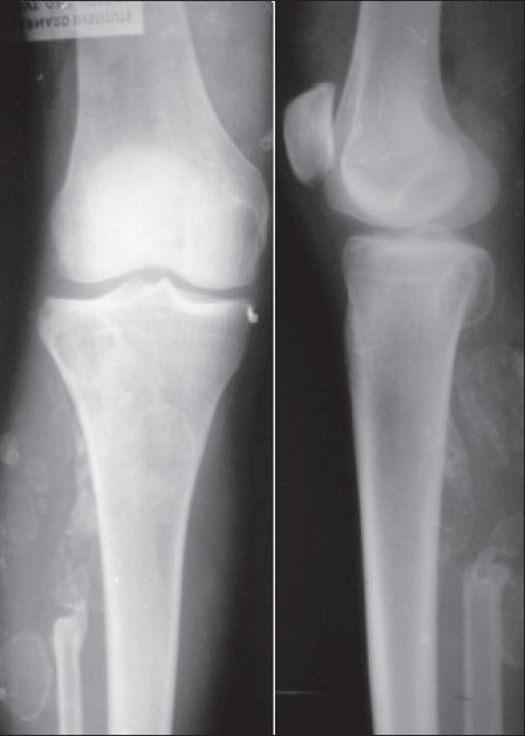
Plain X-ray (AP and lateral view) showing multiple radio opaque septate rim calcified lesions in soft tissue.
